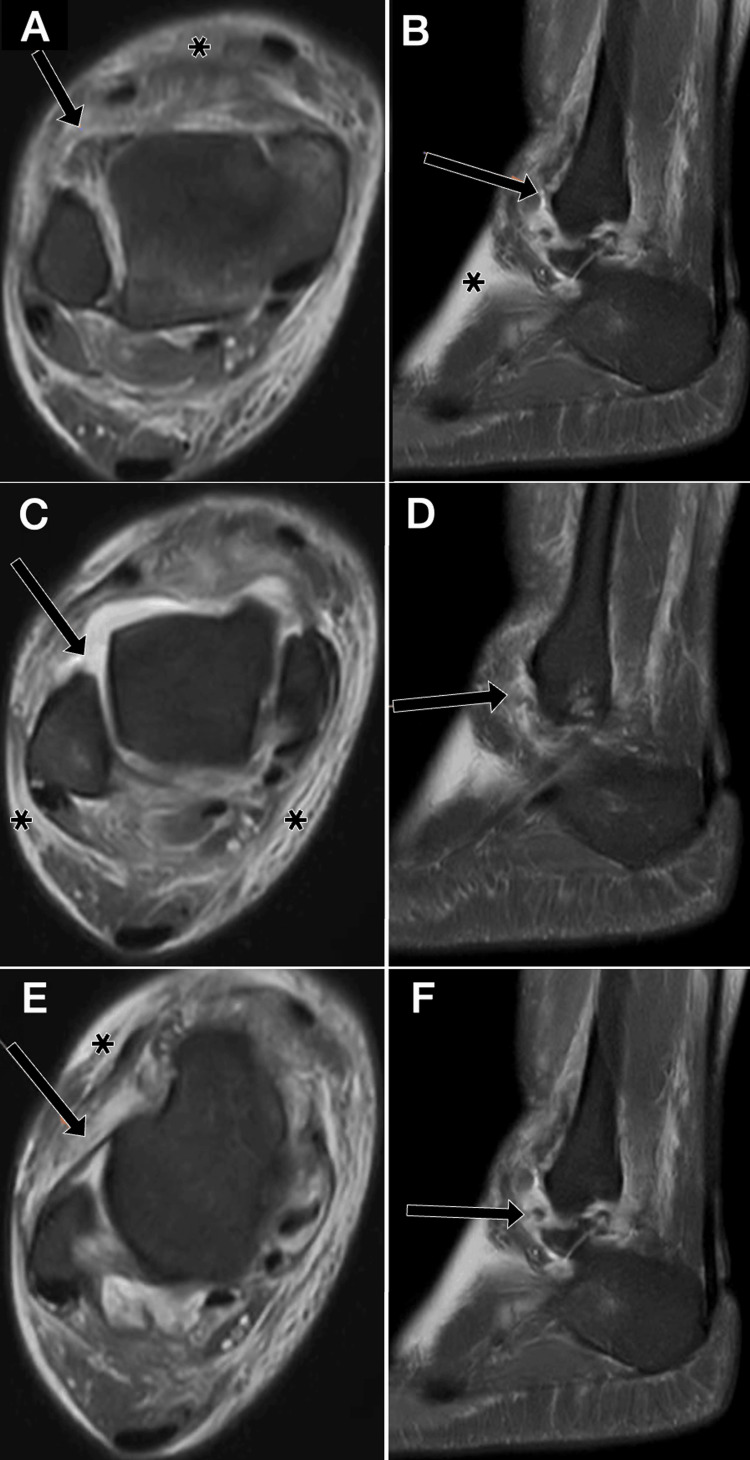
Syndesmotic and anterior talofibular ligament injuries following inversion trauma in a 37-year-old male (DPFS MRI). (A) Axial image at the level of the distal tibiofibular syndesmosis showing a tear of the anterior tibiofibular ligament (black arrow). (B) Sagittal image through the fibula demonstrating the anterior tibiofibular ligament (black arrow) with adjacent soft-tissue edema (*). (C) Axial image at the proximal talus showing a complete tear of the superior fibers of the anterior talofibular ligament (ATFL) (black arrow) with surrounding soft-tissue edema (*). (D) Sagittal image at the distal fibula showing a tear of the ATFL (black arrow). (E) Axial image demonstrating thinned inferior fibers of the ATFL (black arrow) with periligamentous edema (*). (F) Sagittal image at the distal fibula showing residual ATFL fibers (black arrow) with surrounding edema (*). ATFL: anterior talofibular ligament; DPFS: dual proton fat-saturated sequence Images belong to the authors; patient consent was obtained.
